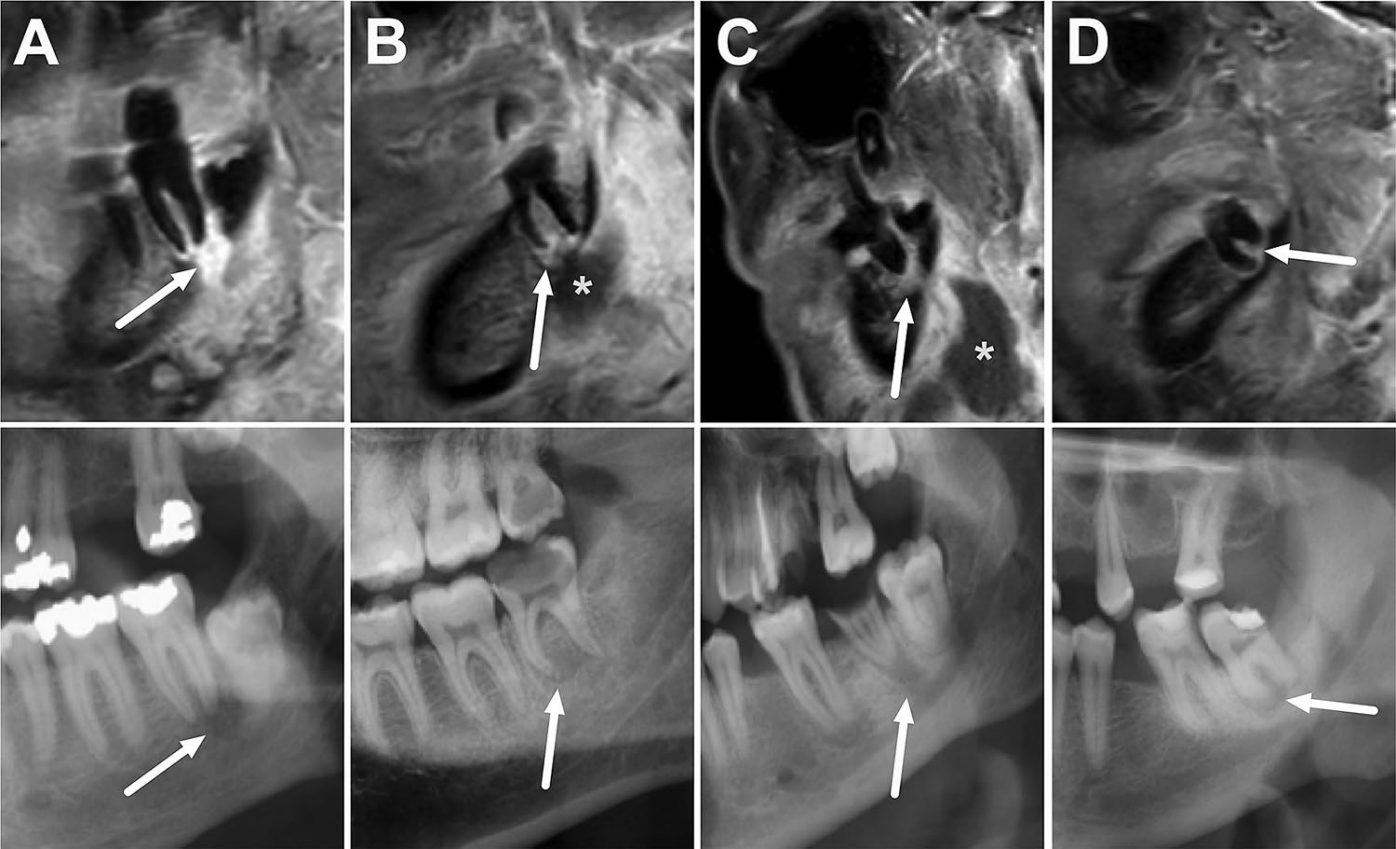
Four patients with odontogenic deep neck infection, periapical infection on T1 fat-suppressed sagittal images with gadolinium (upper row), and corresponding X-ray findings (lower row). Arrows indicate enhancement around the roots (A d47; B d48; C d37; D d38) and periapical lucency on X-ray. Asterisks in (B) and (C) demonstrate adjacent extraosseous abscesses. X-ray images from patients (A) and (B) have been flipped left–right for visualization purposes
